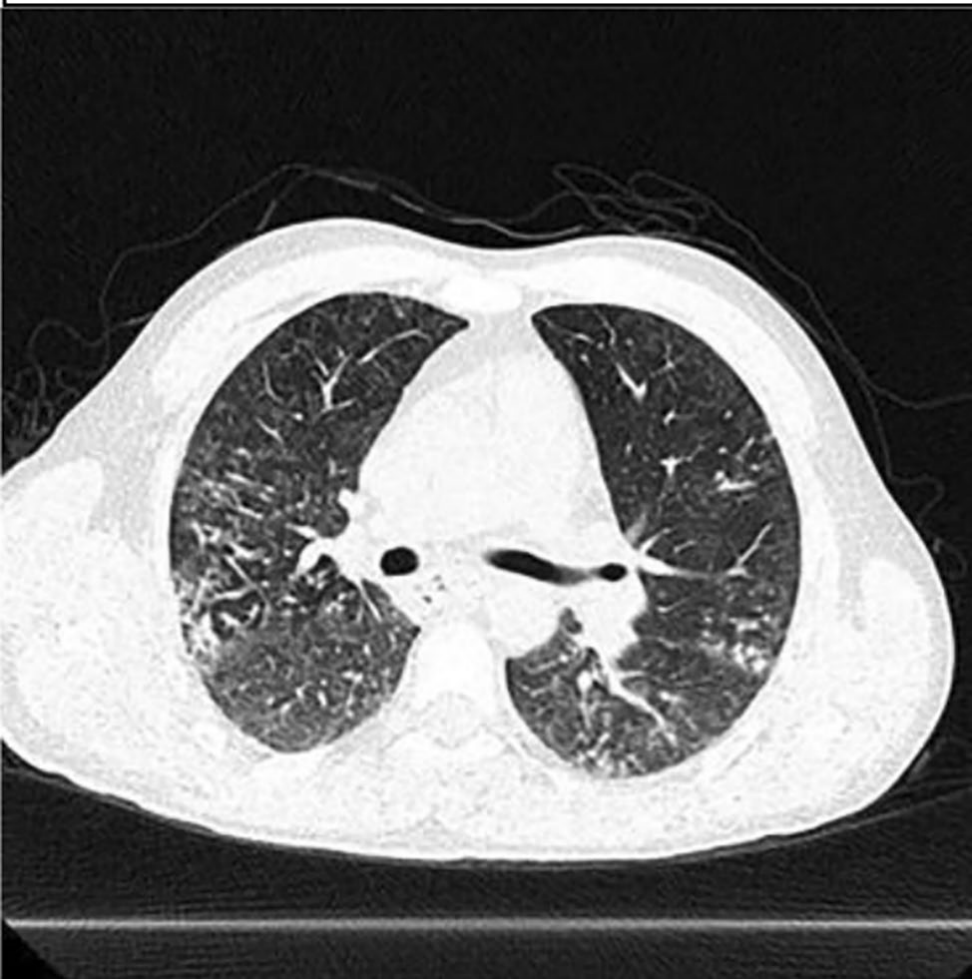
Axial CT image demonstrates nodules in peripheral aspects of right upper and left upper lobes
